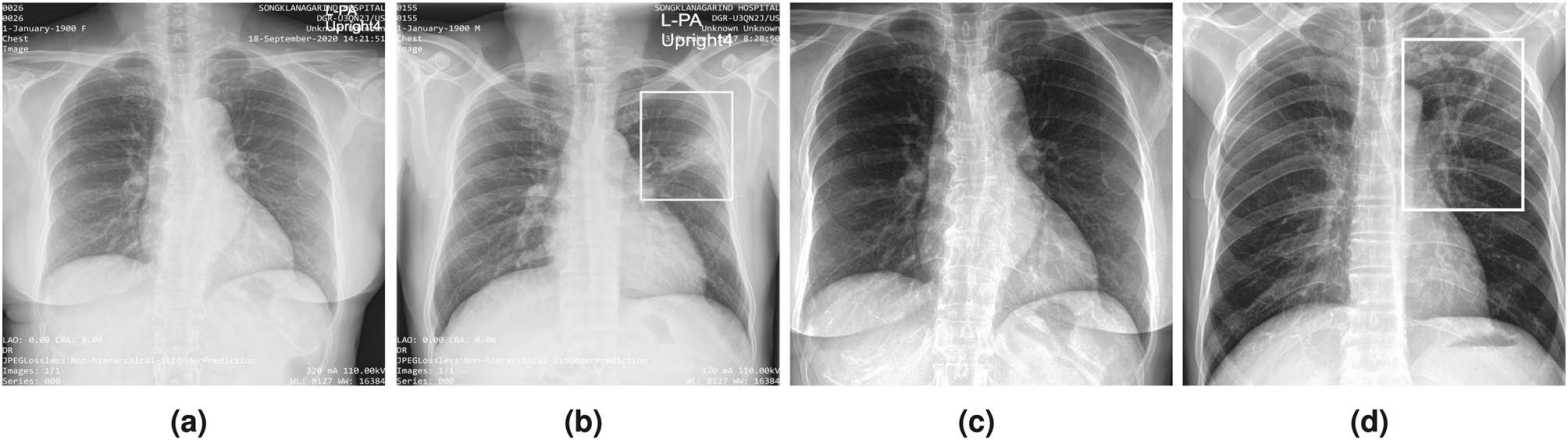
Example of chest X-rays (CXR). (a) Original CXR of a normal case; (b) Original CXR with active pulmonary tuberculosis. The white rectangle denotes reticulonodular infiltration; (c) Pre-processed CXR with normal findings; (d) Pre-processed CXR with active pulmonary tuberculosis. The white rectangle denotes reticulonodular infiltration.
